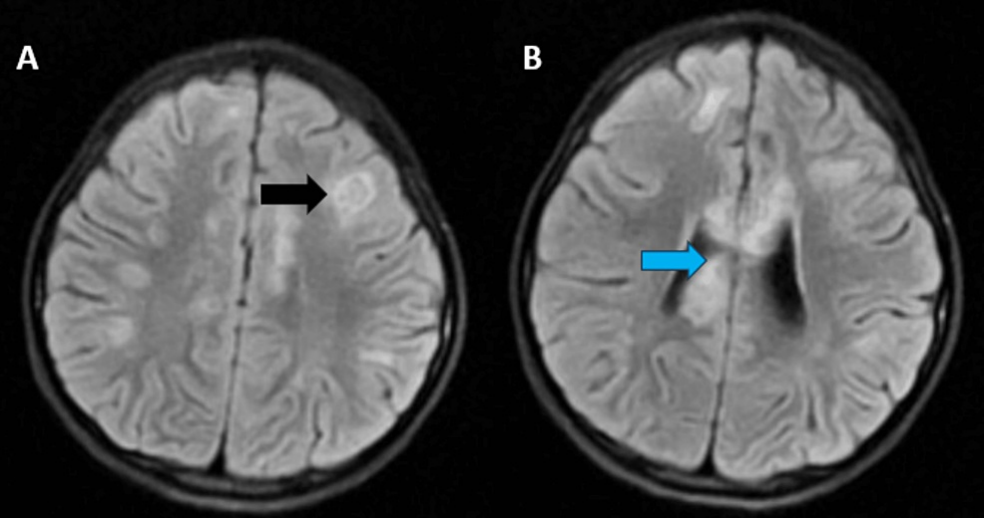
A: FLAIR sequence showing hypointensities in the subcortical white matter (black). B: FLAIR sequence showing the lesions involving the corpus callosum (blue). FLAIR: fluid-attenuated inversion recovery
